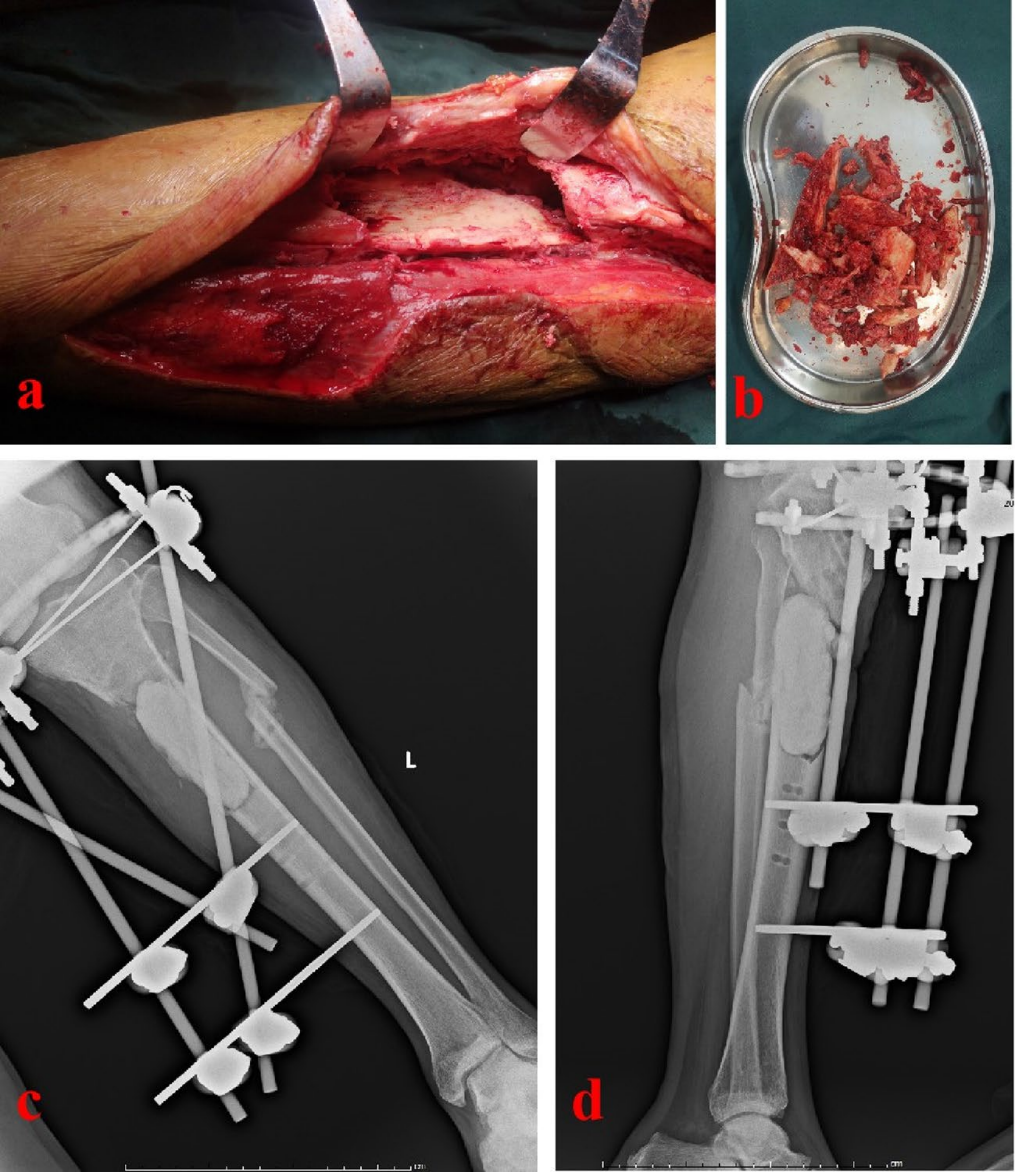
Radical debridement and external fixation were performed for this patient. (a) The intraoperative picture showed the status after removal of all sequestrum; (b) The tray showed the sequestrum removed during operation; (c) and (d) X-ray films after debridement showed that, the semicylindrical tibial defect site (about 8.0 cm) was filled with bone cement.
